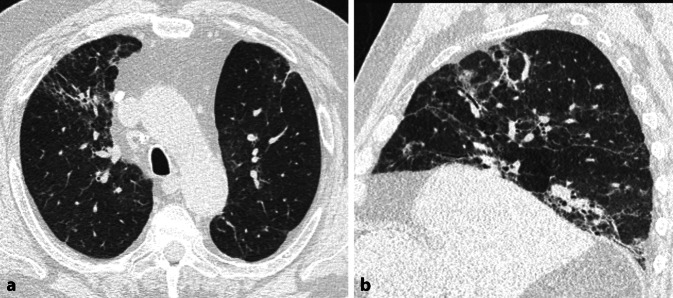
Fibrotische exogen-allergische Alveolitis (EAA) ohne Inflammation. a Axiale und b sagittale multiplanare Reformation (MPR) einer nativen High-Resolution-Computertomographie (HRCT). Fibrose erkenntlich an irreguläre Retikulationen und Traktionsbronchiektasen. Zudem Air-Trapping. Zeichen einer Inflammation zeigen sich nicht
